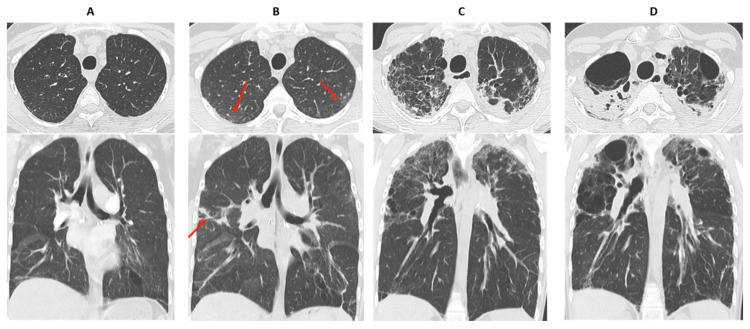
Early detection and evolution of RAS in a 24-year-old man, who underwent bilateral LT in 2007 for cystic fibrosis. Axial and coronal images of CT scans at optimal spirometry (A) 4 months before the diagnosis of CLAD (B), 8 (C) and 22 months (D) after the diagnosis of CLAD. (A) May 2008: normal CT scan; (B) August 2008: first visualization of subtle subpleural ground glass areas and consolidation (red arrows), considered nonspecific and treated with antibiotics. The diagnosis of CLAD was confirmed in December 2008 with a RAS profile; (C) August 2009: rapid evolution towards destructive, upper predominant cystic lesions, and interstitial fibrosis; (D) October 2010: late RAS. CT changes suggestive of pleuroparenchymal fibroelastosis with dense subpleural fibrosis and pleural thickening; dramatic loss of volume of the upper lobes.
